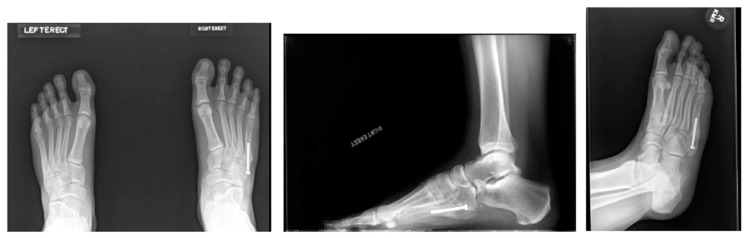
Weight-bearing AP, lateral, and oblique radiographs of Patient 1 (21-year-old male) after IM screw fixation.
